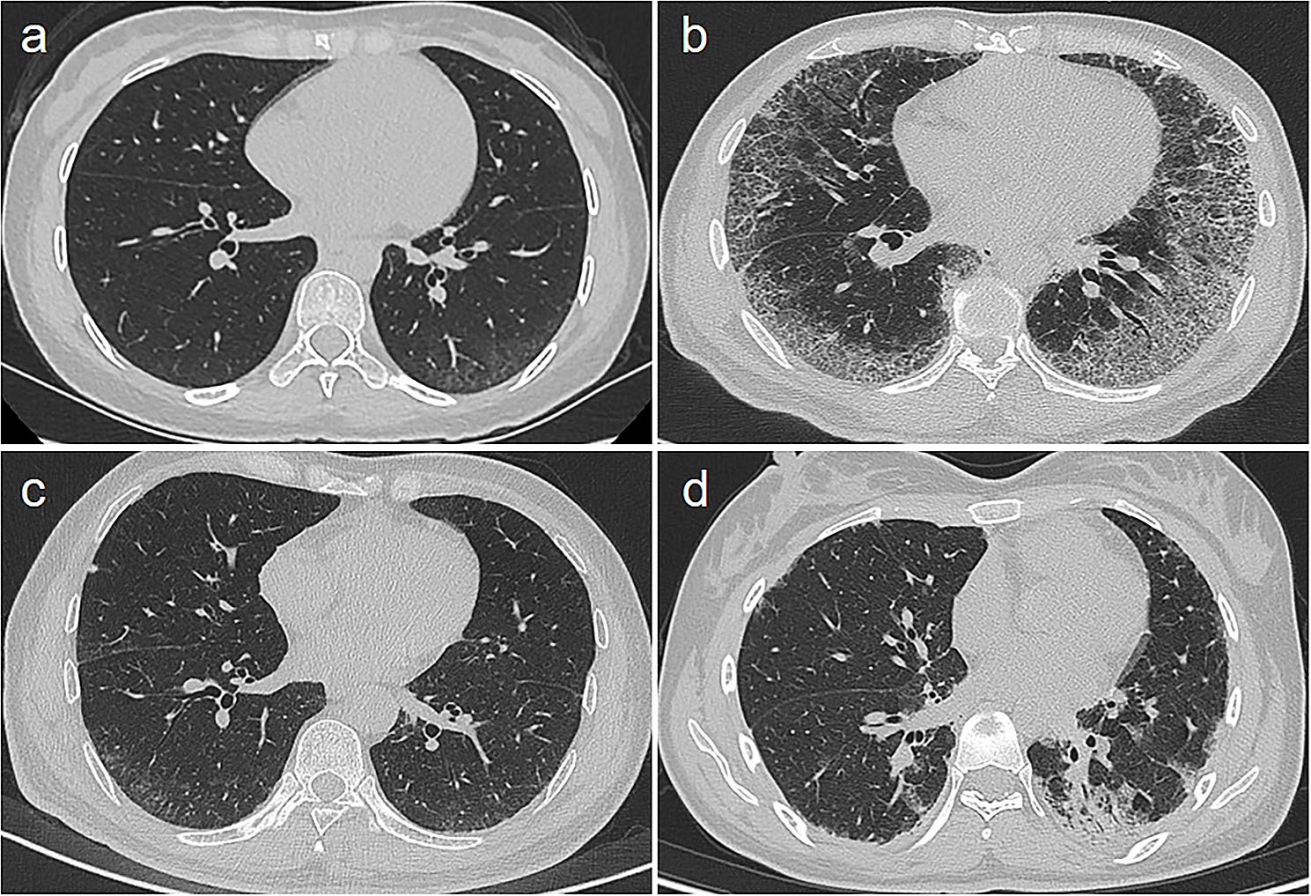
HRCT findings in anti-MDA5 + DM-ILD patients with or without RP-ILD in the external validation dataset. (a) A 49-year-old male patient developed RP-ILD with a Risk-score of 0.6792 and mild pulmonary findings on HRCT; (b) A 43-year-old male patient developed RP-ILD with a Risk-score of 0.7363 and marked interstitial pulmonary fibrosis on HRCT; (c) A 27-year-old female patient didn’t develop RP-ILD with a Risk-score of 0.0647 and mild pulmonary findings on HRCT; (d) A 22-year-old female patient didn’t develop RP-ILD, with a Risk-score of 0.1684 and significant consolidation on HRCT. The optimal cut-off value of the Risk-score for predicting RP-ILD is 0.3140. Note: HRCT, high-resolution computed tomography; RP-ILD, rapidly progressive interstitial lung disease
